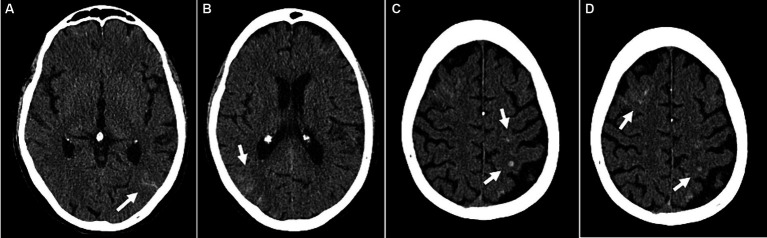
Example of cerebrovascular complications (patient 47): Disseminated small subarachnoid hemorrhages (A,B). Small intracranial hemorrhages in the left parietal and right frontal lobes with adjacent edema (C,D). (A–D) Transversal CT images.
